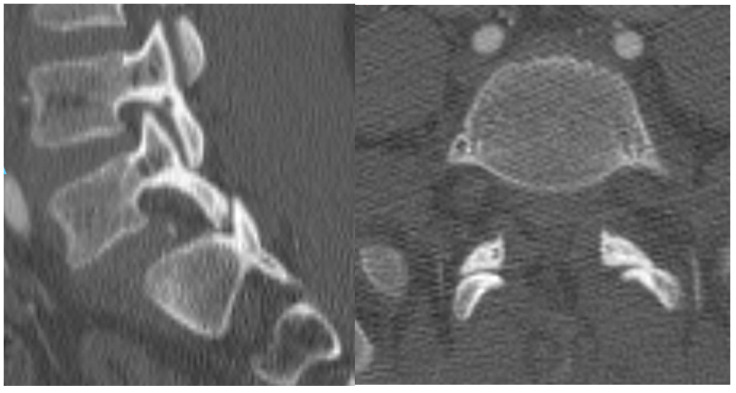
CT scan showing bilateral jumped facet.
